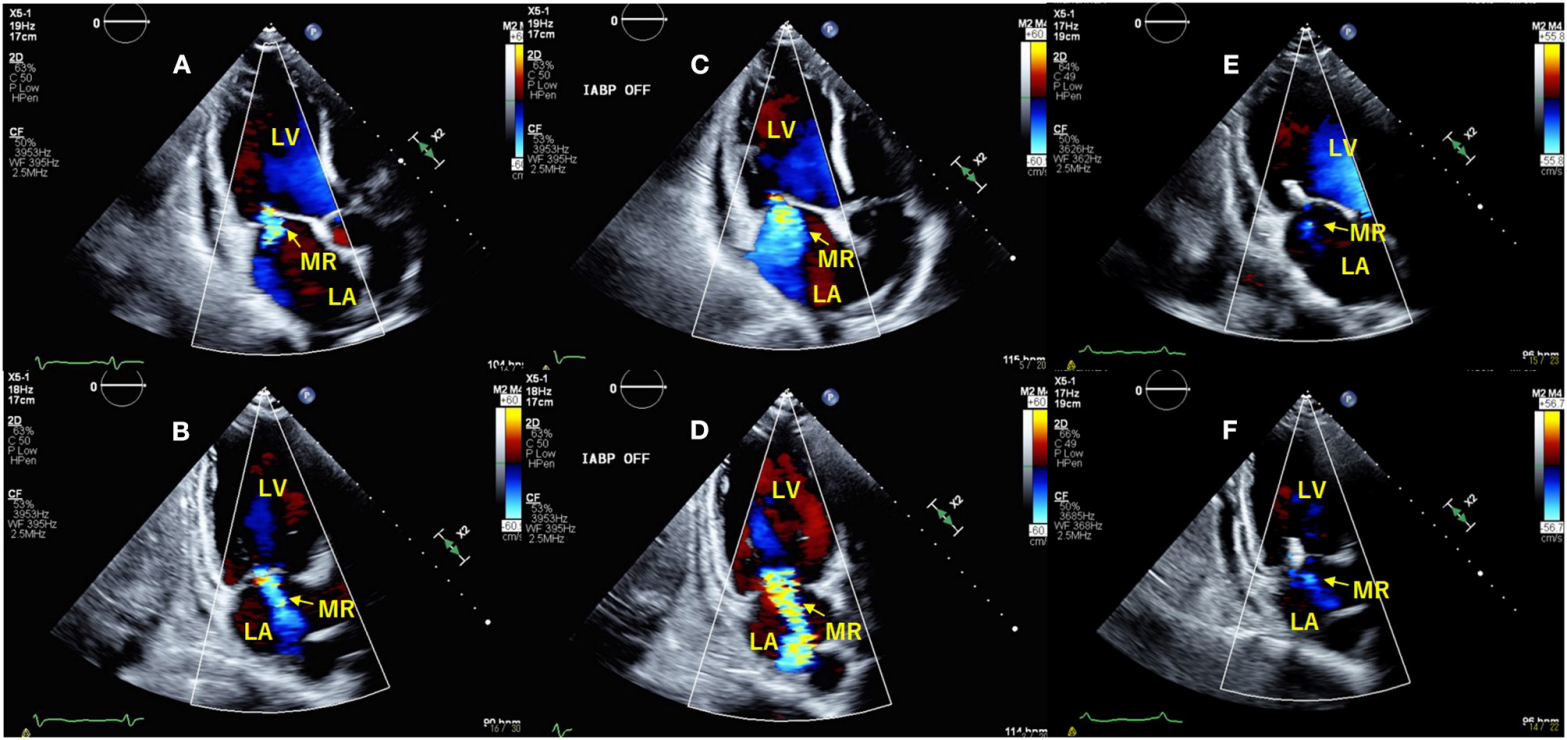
Transthoracic echocardiography with intra-aortic balloon pump (IABP)-ON (A,B) and -OFF (C,D). The apical long-axis view (A,C) and two-chamber view (B,D) show that mitral regurgitation is significantly increased from IABP-ON to IABP-OFF. After intervention with the MitraClip™, secondary MR was significantly decreased (E,F). LV, left ventricle; LA, left atrium.
